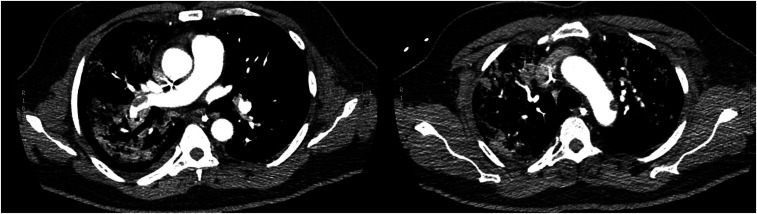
Axial computed tomography pulmonary angiography performed on day 17 after the onset of symptoms. This patient presented with thrombi in both branches of pulmonary artery and aortic arch thrombosis.
